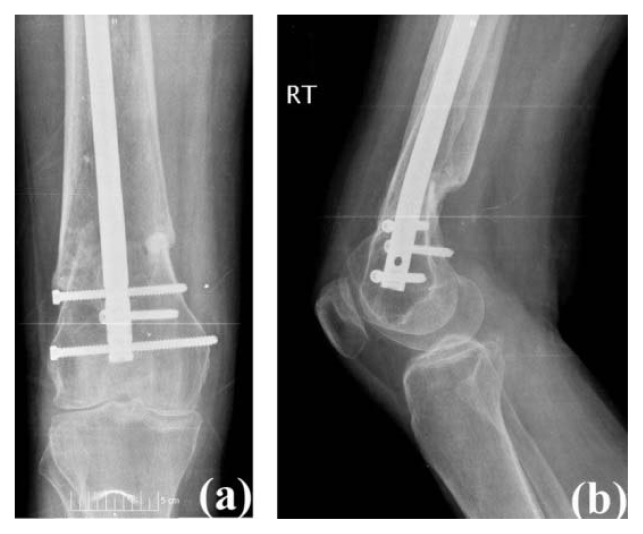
Case #2 pre-operative radiographs: (a) anterior posterior view and (b) lateral view.
